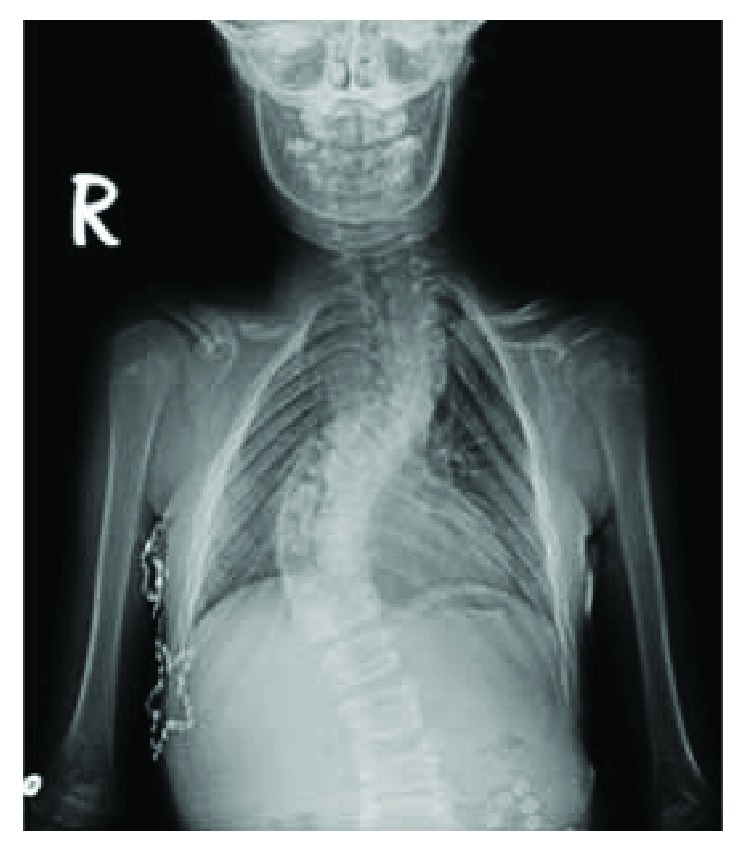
Radiograph of the same bilateral clavicular hypoplasia patient at age 11, showing poor growth of the middle 1/3 of the right clavicle and the absence of the lateral end of the left clavicle. Narrowing of the chest can be seen.
